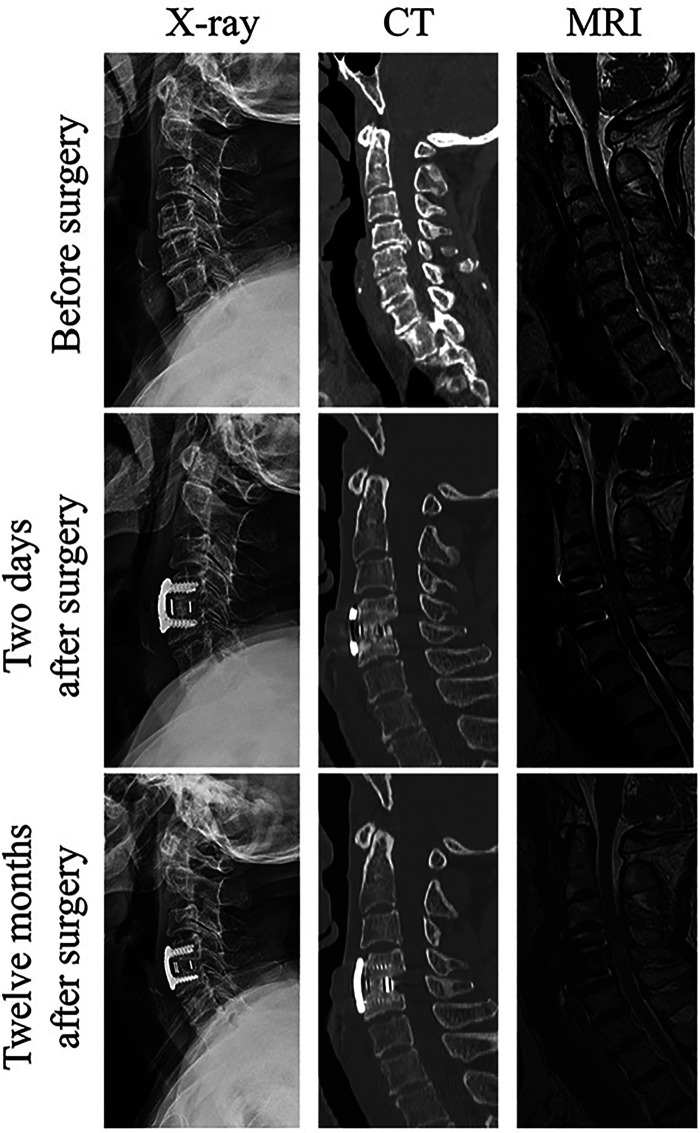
The imageological examination of a 71-year-old female typical case before and after surgery and at the last follow-up. Continuous trabecular bone crossing the cage was observed in the mid-sagittal CT view at the 12-month follow-up, confirming interbody fusion.
